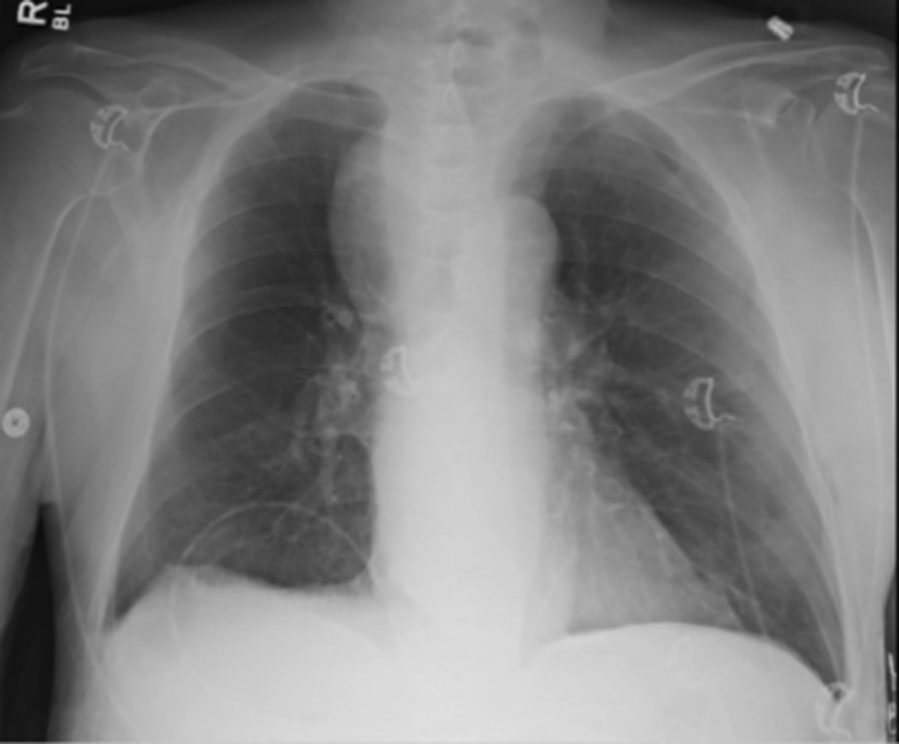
The patient’s chest X-ray showing widened mediastinum. Formal read: large curvilinear soft tissue mass. Convex outward into the right upper lobe arising from the mediastinum. Vascular etiology is considered. Small right pleural effusion. CT is recommended if this is not a known finding from previous studies
